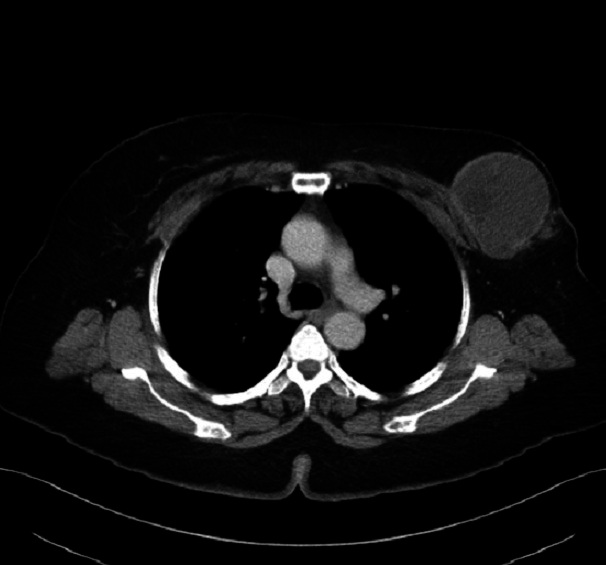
Axial CT image shows left breast mass with internal septations and peripheral enhancement
